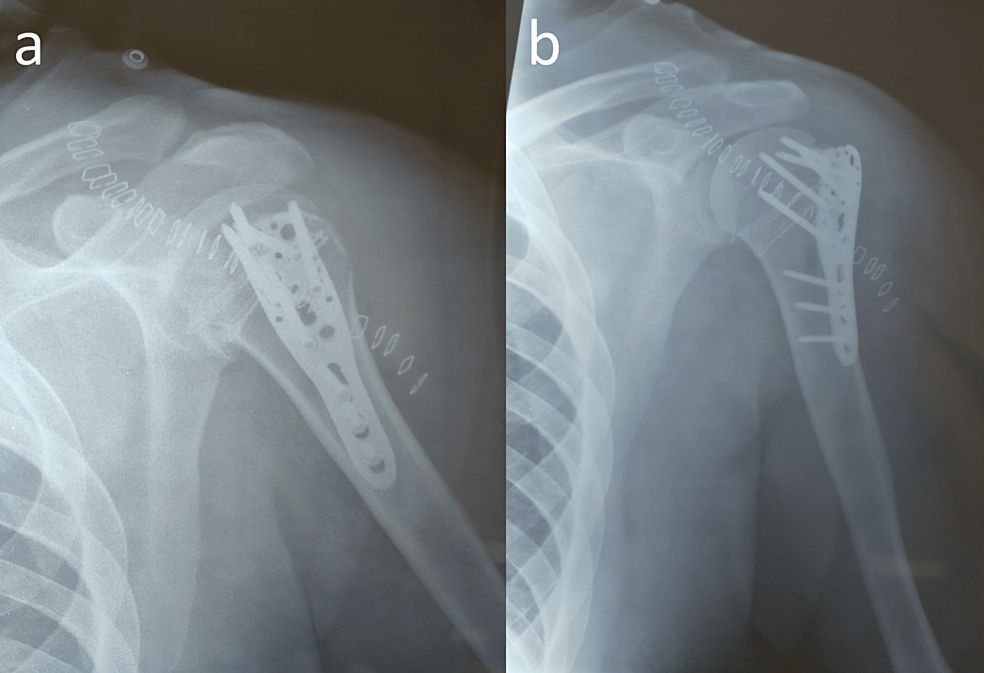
Anteroposterior radiographs of the shoulder with the anatomic plate applied. (a) Radiograph with the arm in internal rotation. The shoulder is reduced and the tuberosities are fixed. (b) Radiograph in external rotation with the arm in external rotation. Both tuberosities are anatomically reduced and fixed.
